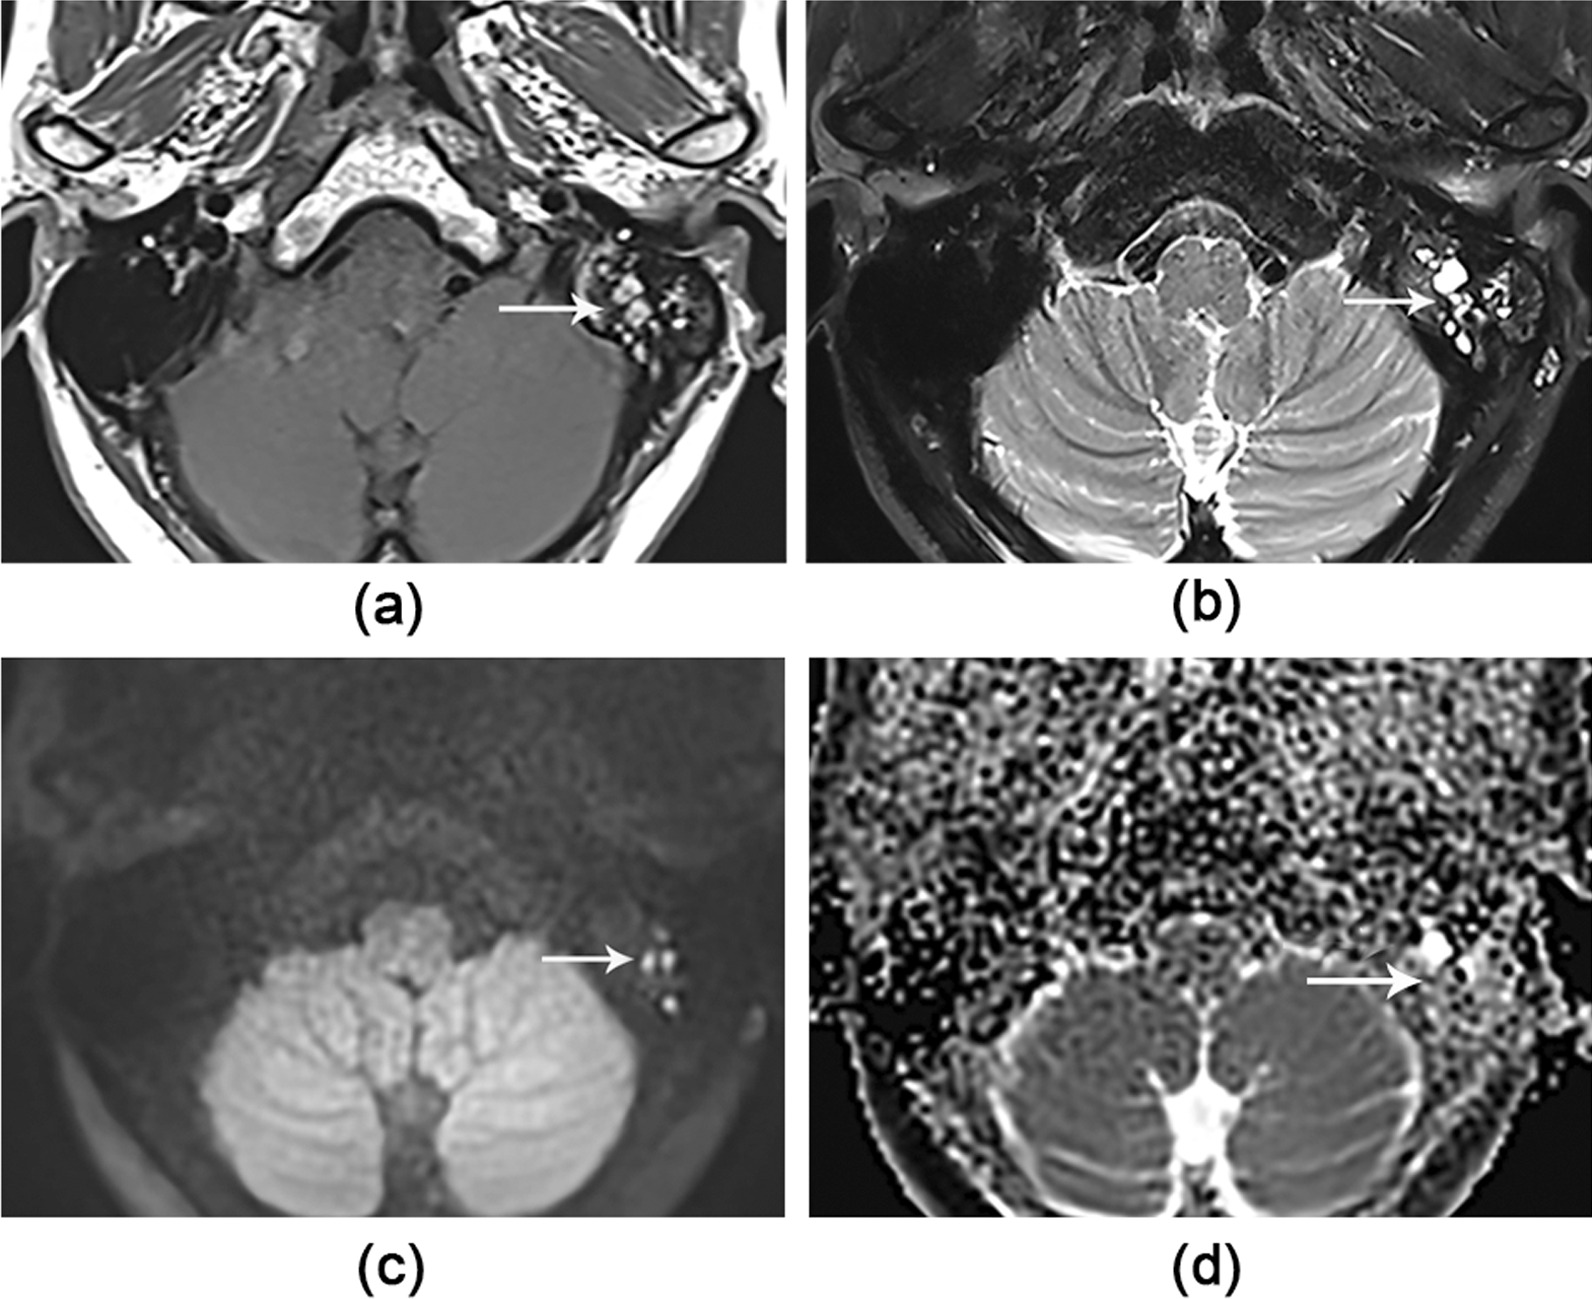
A 52-year-old female patient with false-negative CS in the left side. Axial images show the lesion (white arrow) as high signal on (a) first the T1WI, then (b) T2WI-FS and (c) TGSE BLADE DWI b1000 image, and a low signal on (d) the ADC map
